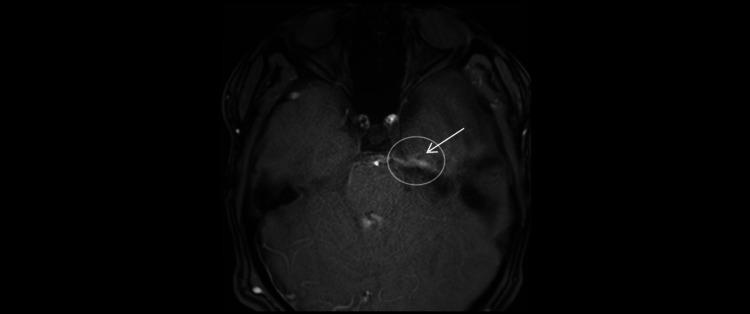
Phase-contrast cine MRI (April 28, 2025; axial 2D PC-MRI; single-slice, 6-8 mm). CSF flow is visualized across the surgical fenestration between the left middle-fossa arachnoid cyst and the basal cisterns (arrow, open circle), confirming patency of the cyst-cistern communication. PC-MRI: Phase Contrast Magnetic Resonance Imaging.
